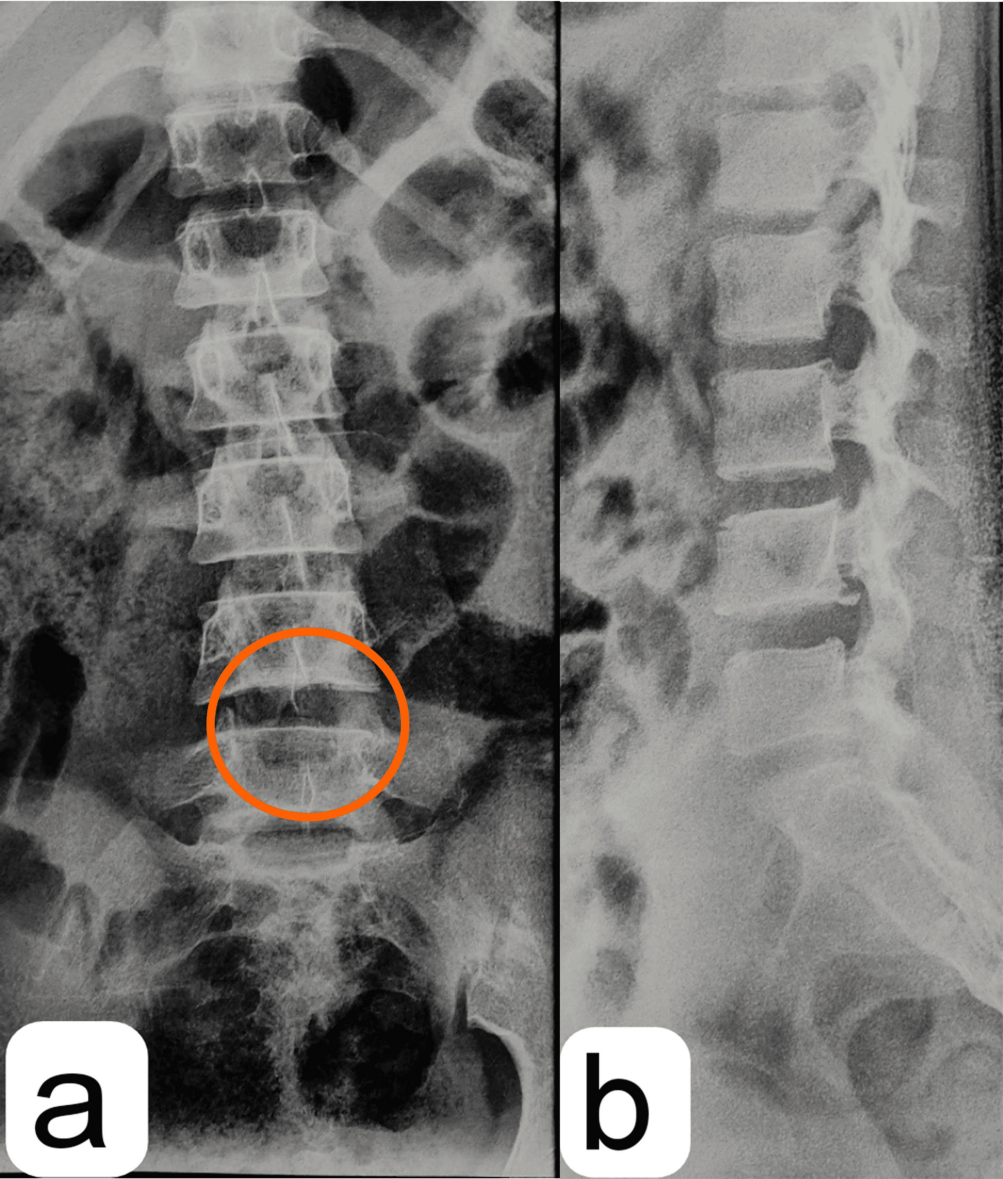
Postoperative X-ray (a) AP and (b) lateral view of the LS spine Postoperative X-ray showing the (a) AP view of the LS spine with the site of laminectomy and bilateral decompression at the L4-L5 level on the left side (marked area) and (b) postoperative X-ray of the LS spine in lateral view. AP: anteroposterior, LS: lumbosacral
